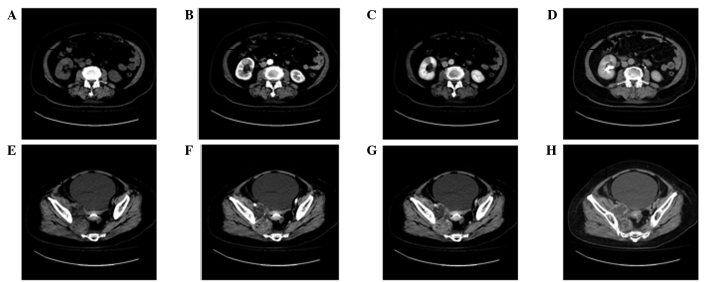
Case 1. Contrast-enhanced CT scans show a low density focus (1.9×2.1 cm) in the right kidney and an iliopsoas abscess. (A and E) Plain scan; (B and F) arterial phase; (C and G) venous phase; and (D and H) delayed phase. CT, computed tomography.
